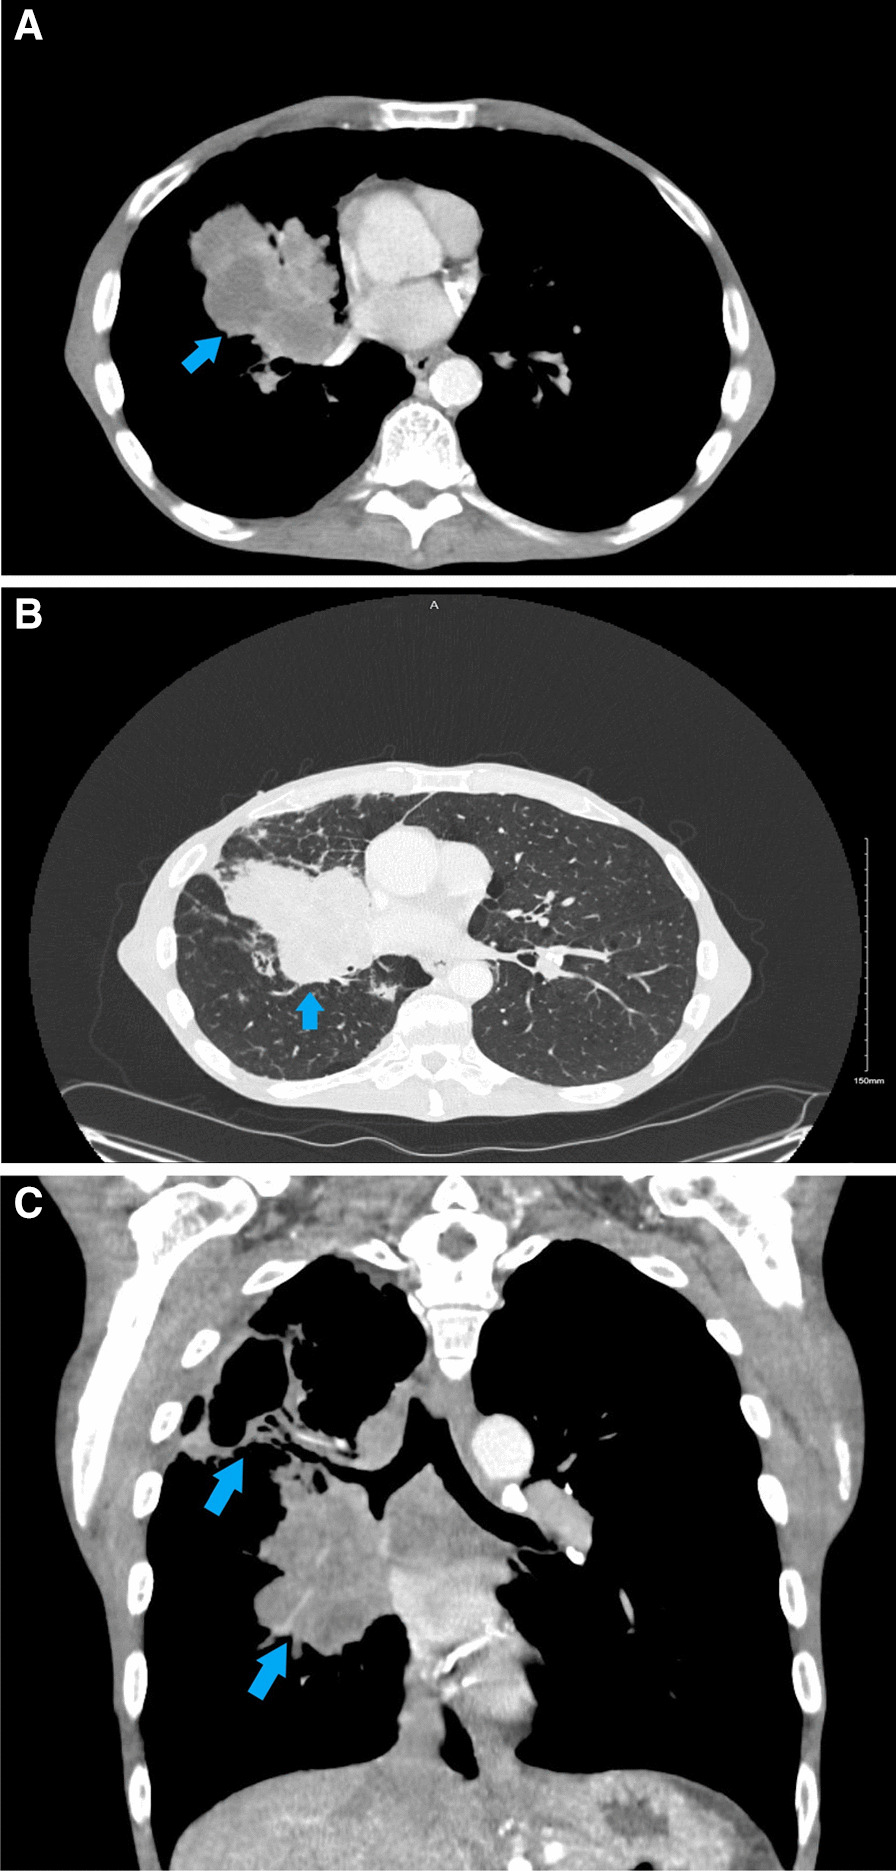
A Trans-axial computerized tomography (CT) Chest showing right upper lung mass (blue arrow). B Trans-axial computerized tomography (CT) Chest in lung window showing right upper lung mass (blue arrow). C Coronal view computerized tomography (CT) chest showing the right upper lobe lung mass (blue arrow). Findings worrisome for extensive malignancy involving the mediastinum, right hilum, and right upper lobe
